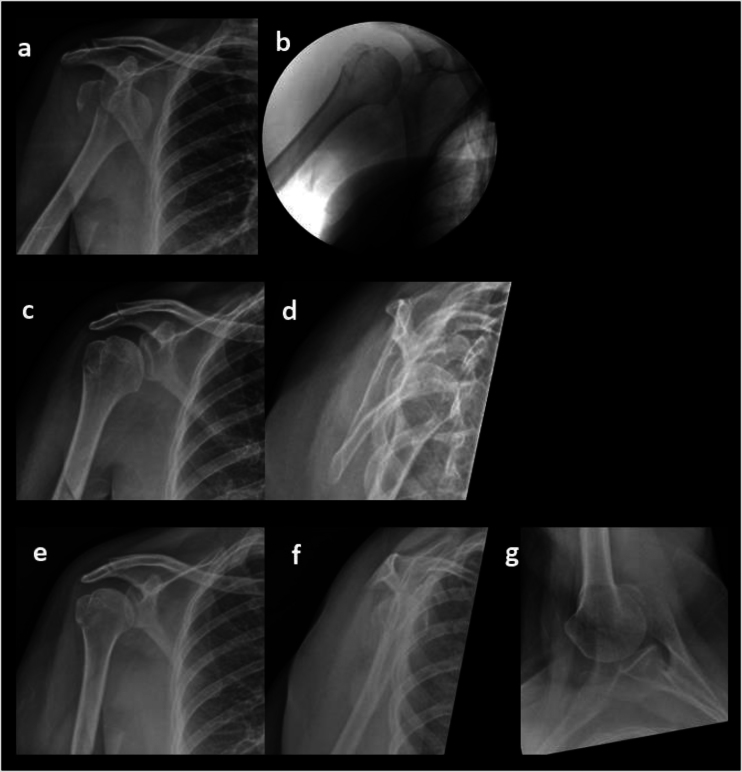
Delayed displacement following greater tuberosity fracture-dislocation. (a) AP radiographs of left shoulder with anterior GT fracture dislocation. (b) Intraoperative AP view following successful closed reduction with minimally displaced GT fragment; (c,d) AP and Y view radiographs showing no displacement of GT fragment at 1 week follow up. (e–g) AP, Y view and axillary view radiographs at 3 weeks showing displaced GT fragment. AP = anteroposterior; GT = greater tuberosity; Y = lateral scapula shoulder
